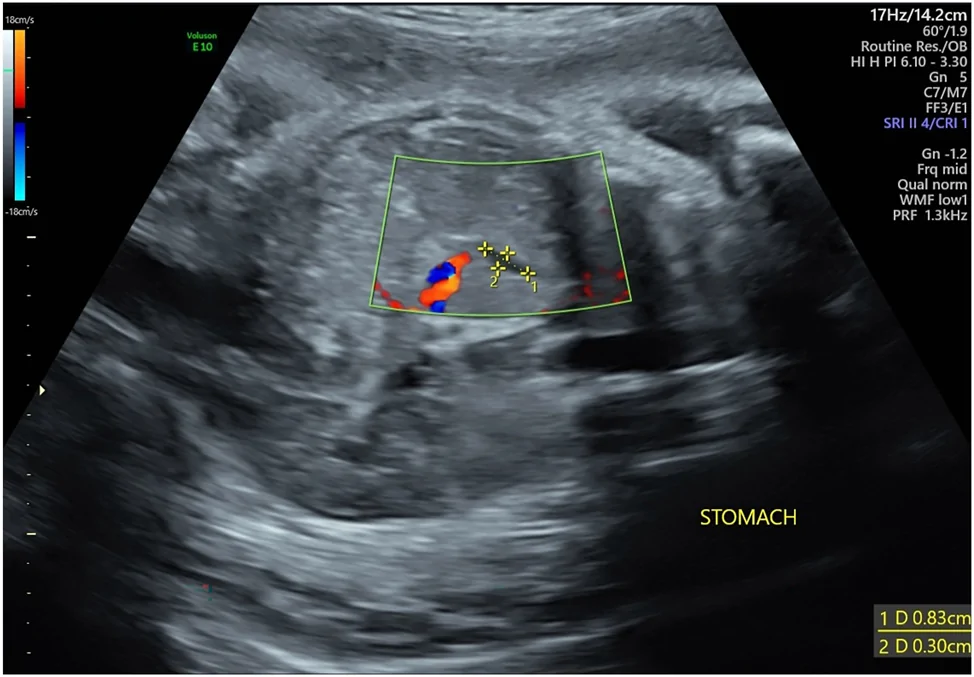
Ultrasound showing small stomach.
